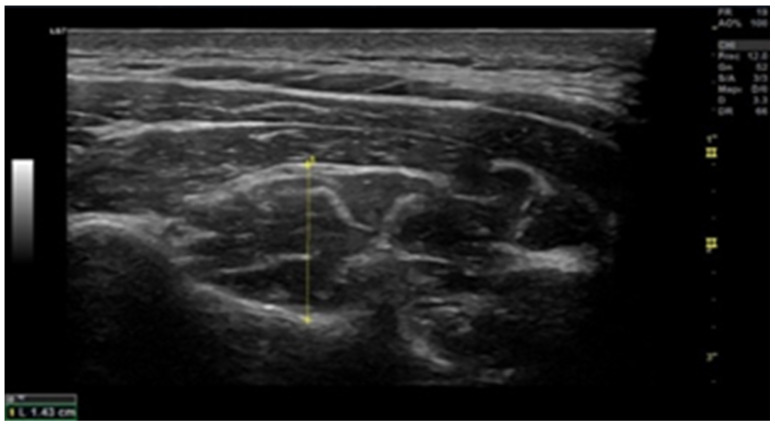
Multifidus ultrasound image at C5 (transverse view). The probe is placed 90° to the lamina of C5 where the rater considered the muscle to be at its thickest and up to the echogenic line of the hyperechoic fascia between the semispinalis cervicis and semispinalis capitis.
